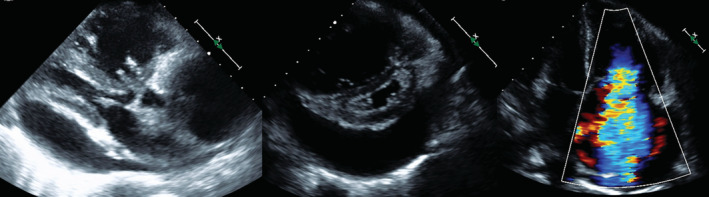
Echocardiography revealing dilatation of the right ventricle, severe tricuspid regurgitation with a flattened interventricular septum, and moderate pericardial effusion.
